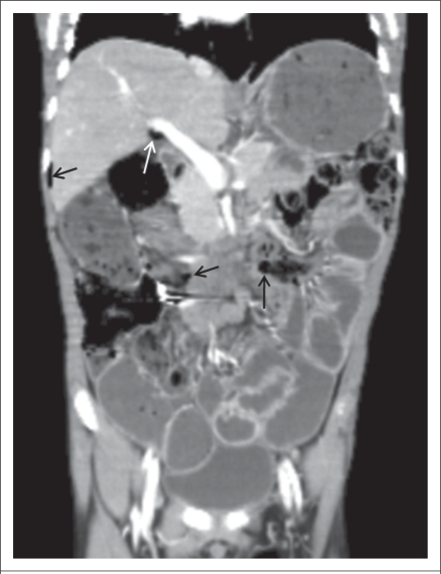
Coronal abdominal CT image showing a little small-bowel pneumatosis, pneuperitoneum (black arrows), and hepatic portal venous gas (white arrow)
